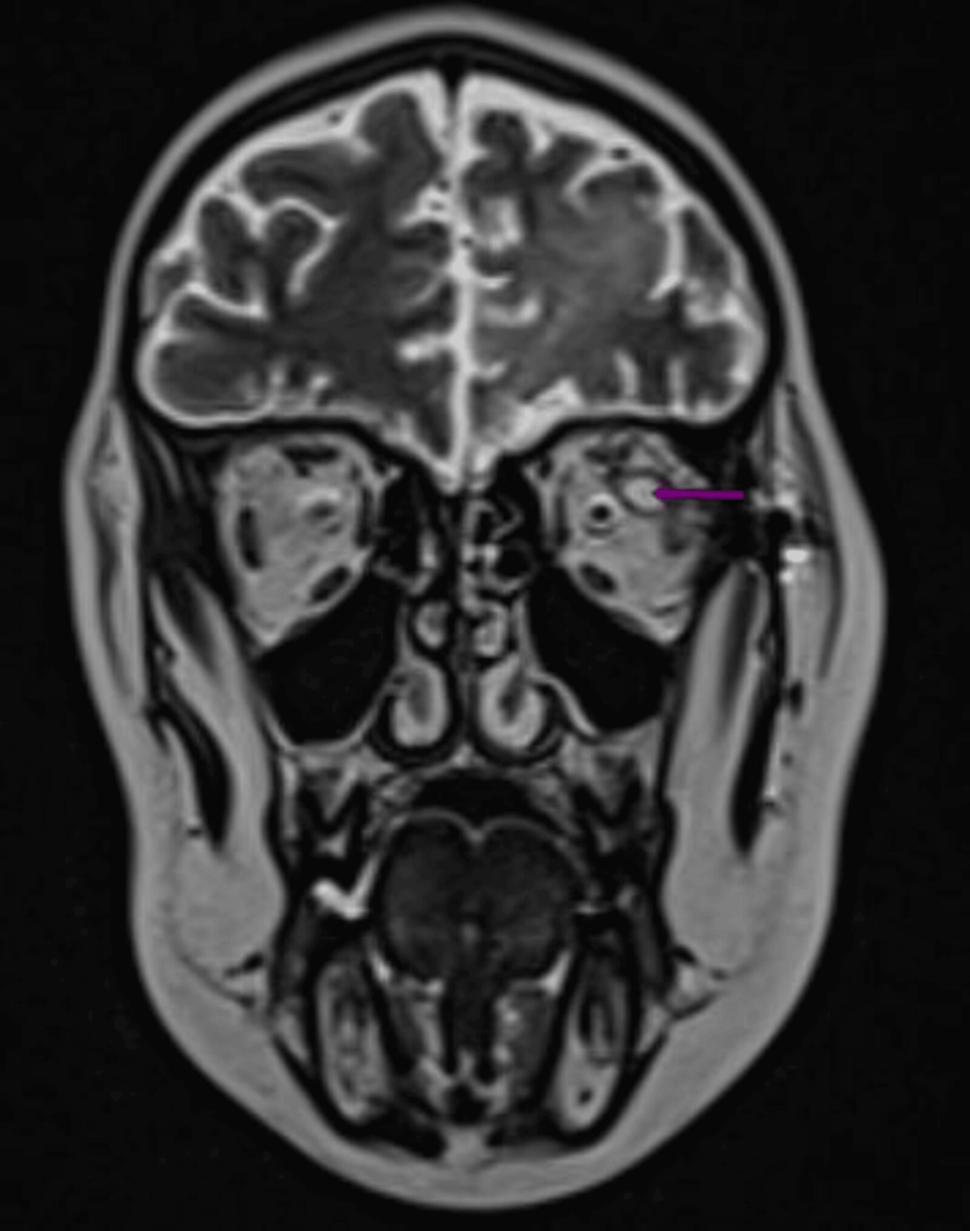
Coronal T2 MRI section revealing a well-circumscribed intraorbital conal mass located at the level of the left superolateral orbital wall, with hypersignal on T2 (purple arrow). MRI, magnetic resonance imaging
